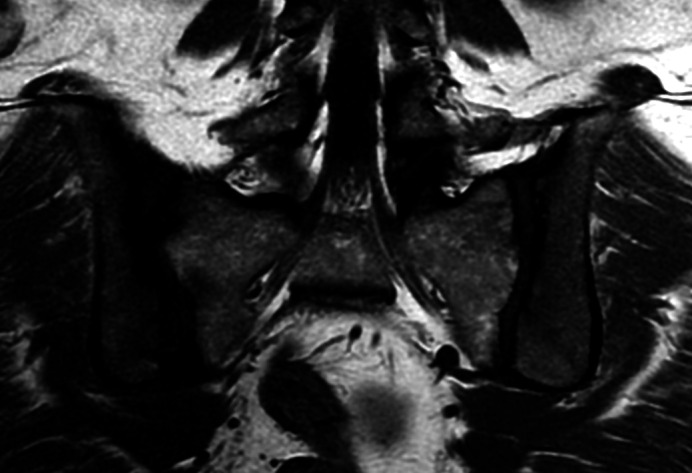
Sacro-iliite droite. Sclérose sous-chondrale (bande hypointense). IRM, image pondérée en T1 Right sacroiliitis. Subchondral sclerosis (hypointense band). MRI T1-weightened image
